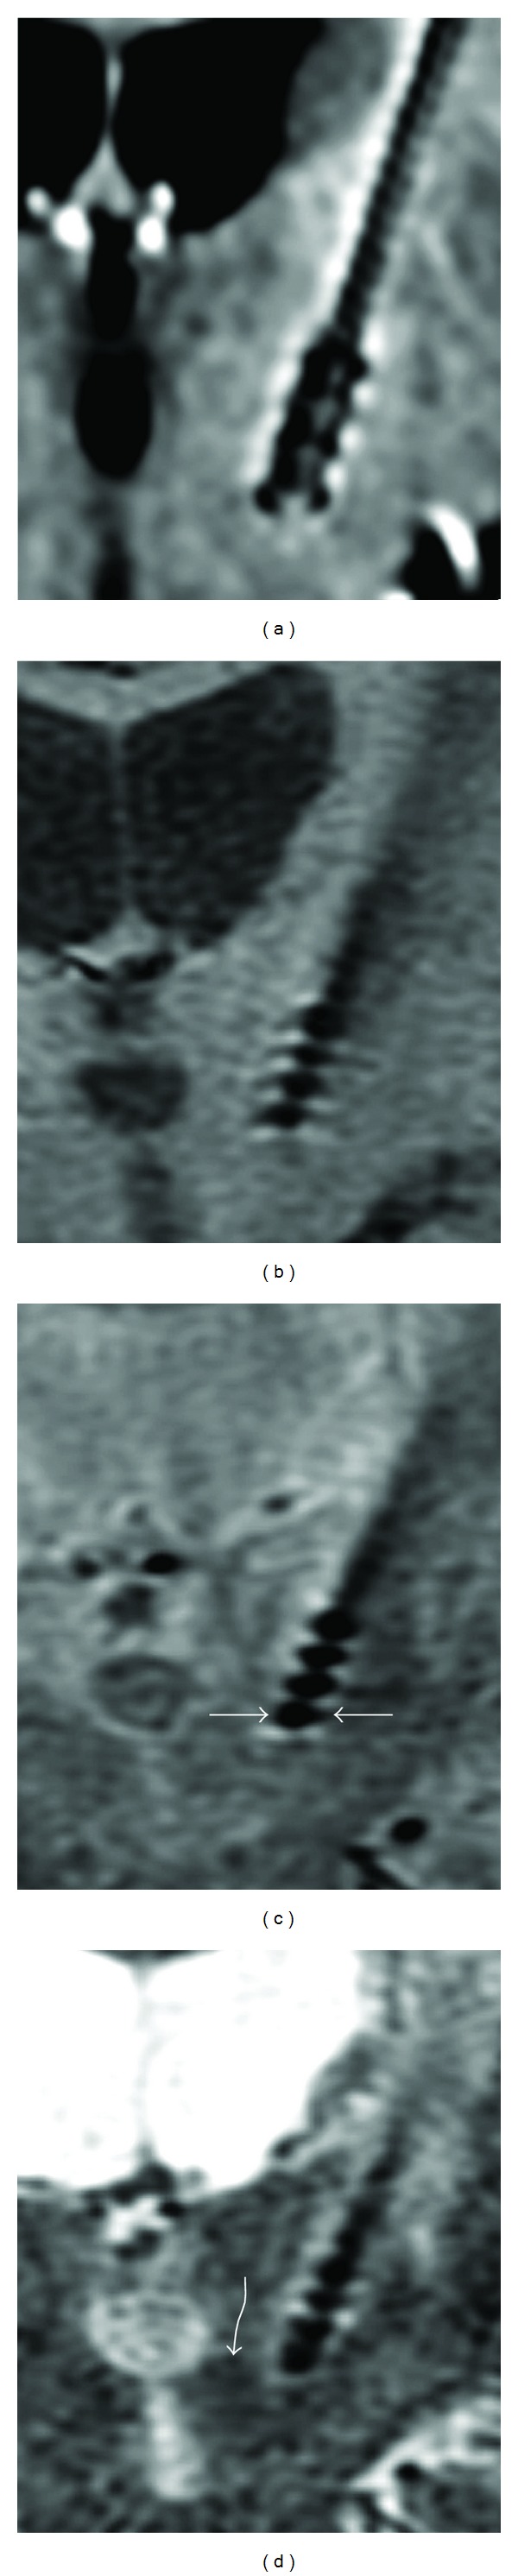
Typical coronal MR images, (a) a section from 3D MPRAGE; measured contact diameter (measurement plan is shown in panel (c) by straight arrows) = 4 mm, more than that from (b) a section from 2D SE T1 (2.2 mm) or (c) 2D FSE PD (2.3 mm) and (d) 2D FSE T2 (2.3 mm) sequences. The location of the lead at left subthalamic nucleus is indirectly estimated as 11-12 mm lateral to midline across the superior-anterior border of the red nucleus (curved arrow, panel (d)).
